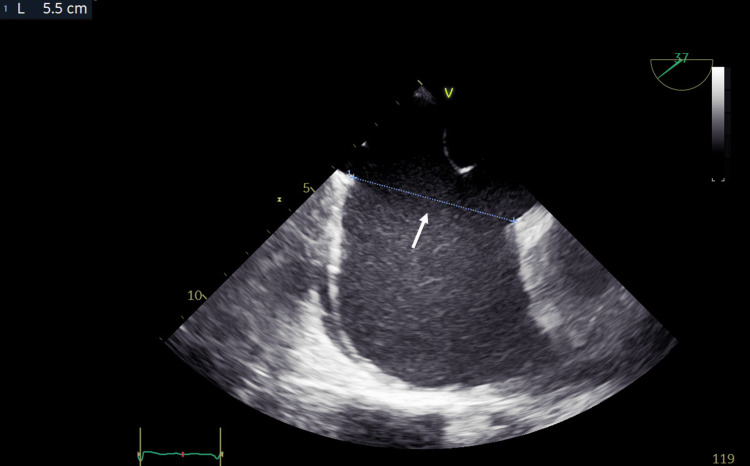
Transesophageal echocardiography (bicaval view) of Case 3 showing a large ostium secundum atrial septal defect (ASD) measuring 55 mm with deficient septal rims (white arrow), precluding percutaneous closure and leading to surgical referral.
